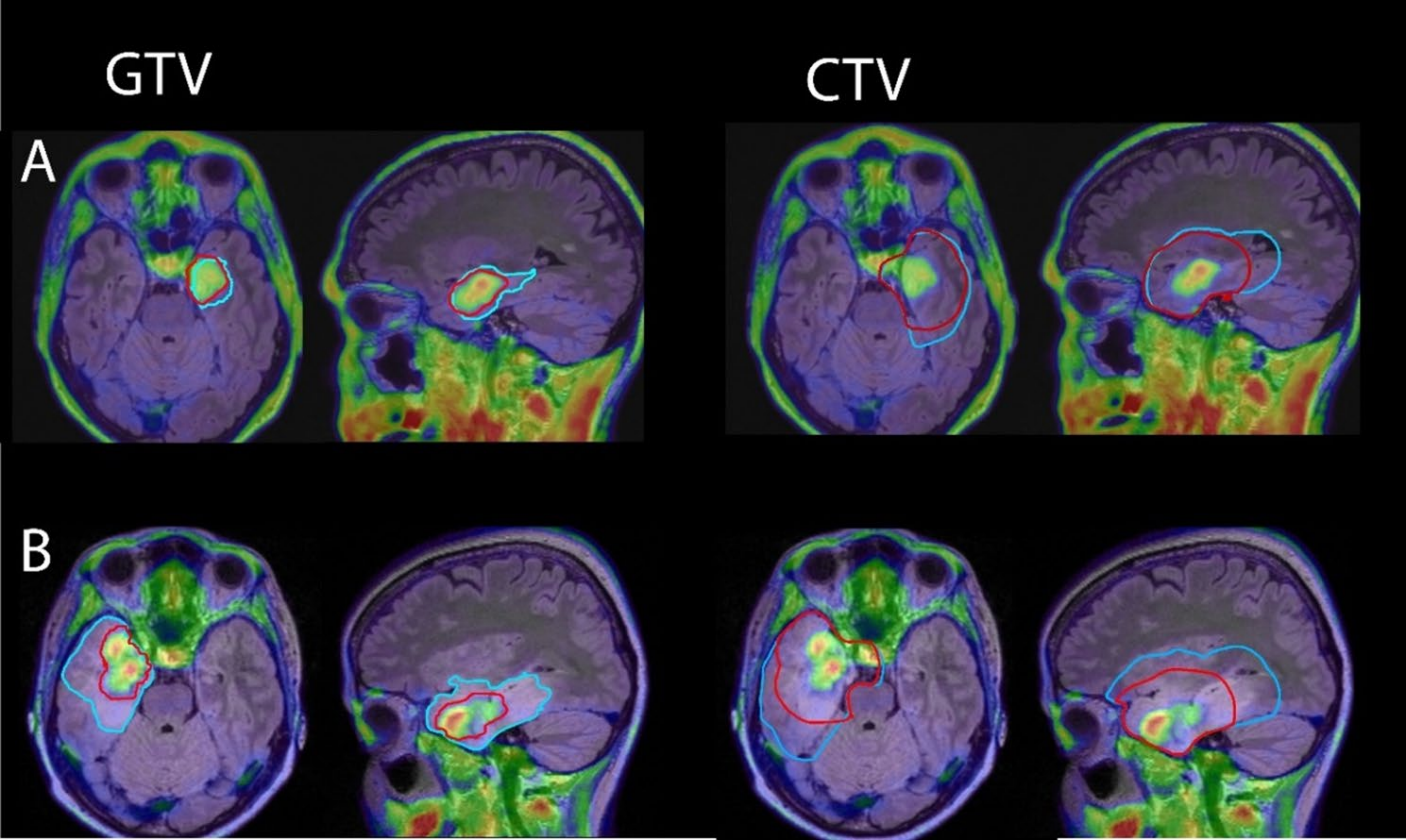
Examples of PET (red) and MR (blue) volumes in two patients with glioblastoma where MR GTVs included FLAIR hyperintensity due to lack of contrast uptake (A) or only partial contrast uptake (B). GTV, gross tumor volume; CTV, clinical target volume
